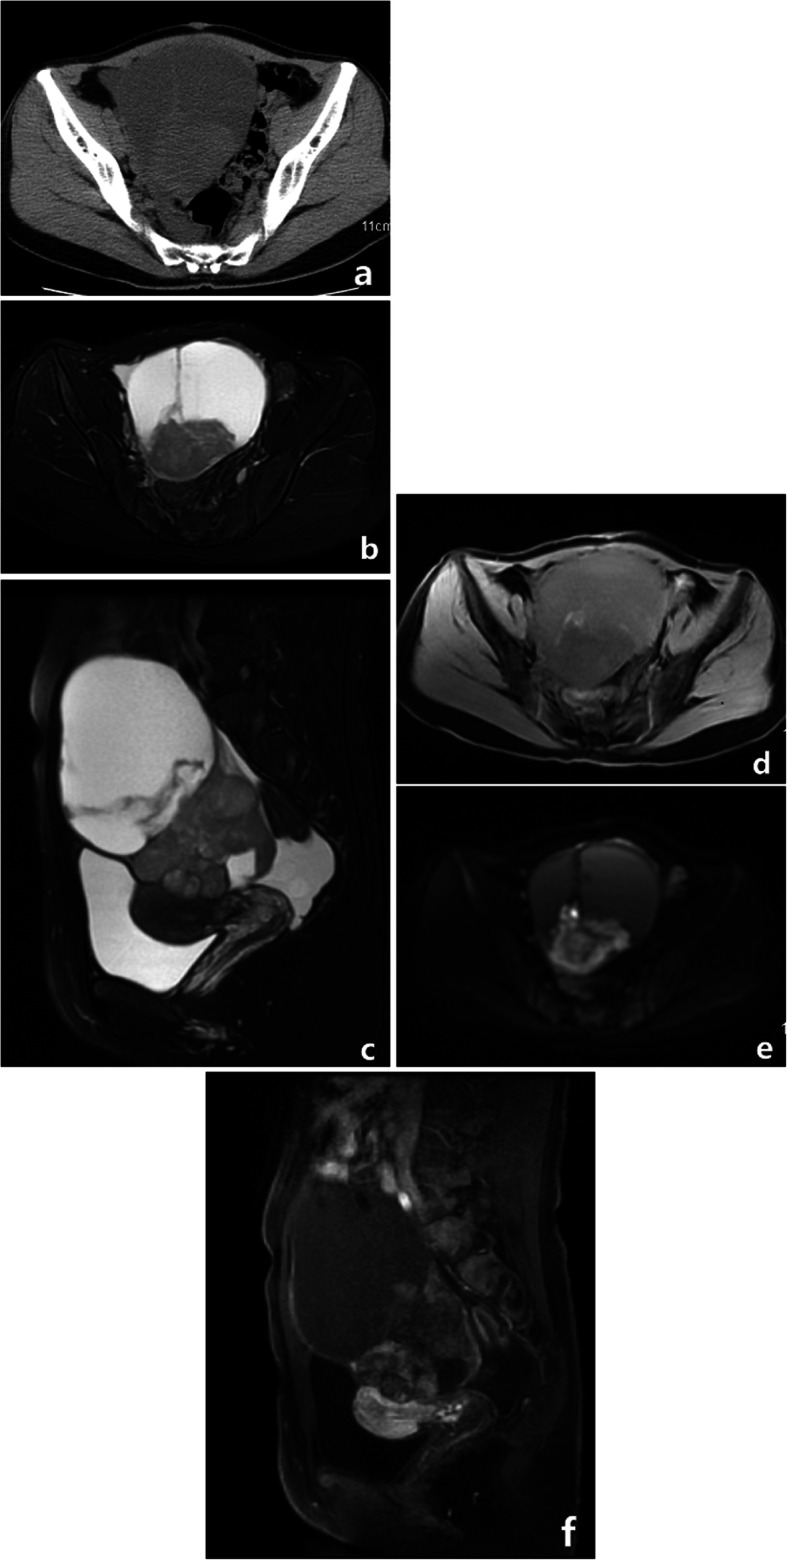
OCS in a 43-year-old woman with abdominal pain. a Axial CT showed a multilocular cystic mass with a large solid protrusion. The solid component was iso-dense on CT and slightly high SI on fat saturation T2WI (b, c), slightly low SI on fat saturation T1WI (d), and high SI on DWI (e), along with hemorrhagic patchy high SI on fat saturation T1WI insider the tumor. f After the administration of contrast agent, the tumor parenchyma can appear heterogeneous moderate enhancement
